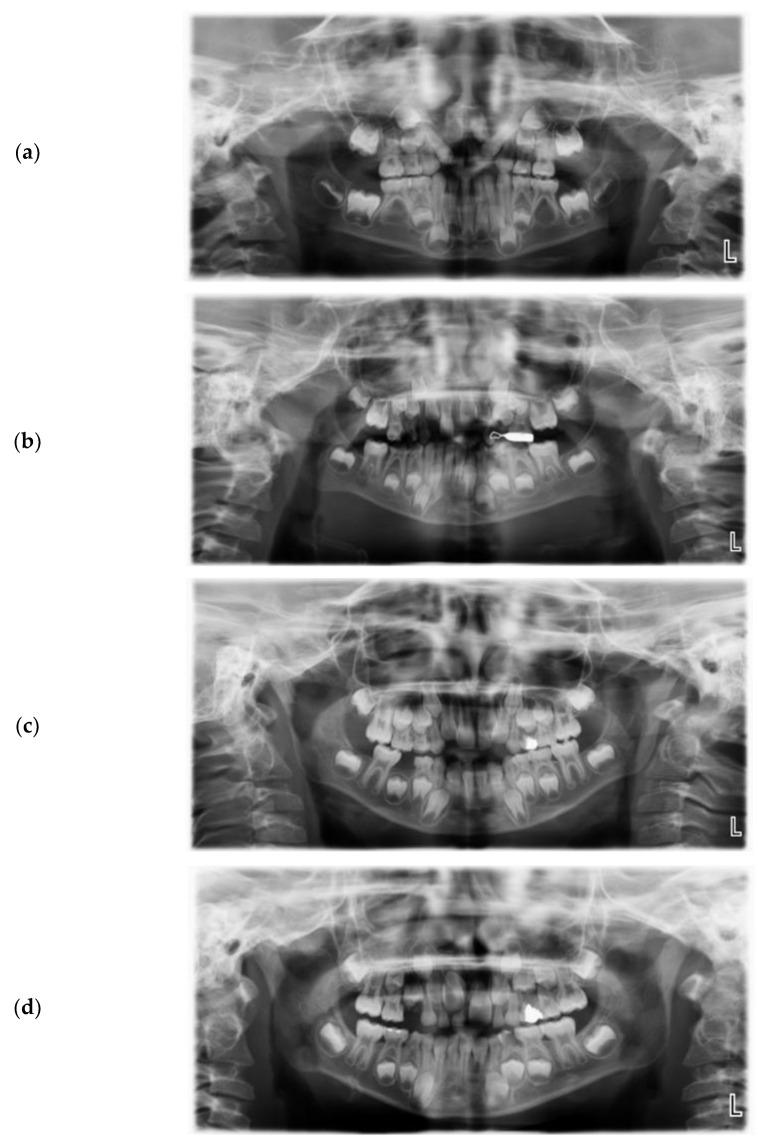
(a) Panoramic radiograph showing delayed eruption of permanent upper central incisor due to cleft lip and palate and insufficient space. (b) Delayed eruption of permanent upper central incisors due to early loss of primary upper central incisors. (c) Delayed eruption of permanent upper central incisors due to retained primary upper left central incisors. (d) Delayed eruption of permanent upper central incisors due to retained primary upper incisors and the presence of supernumerary teeth.
